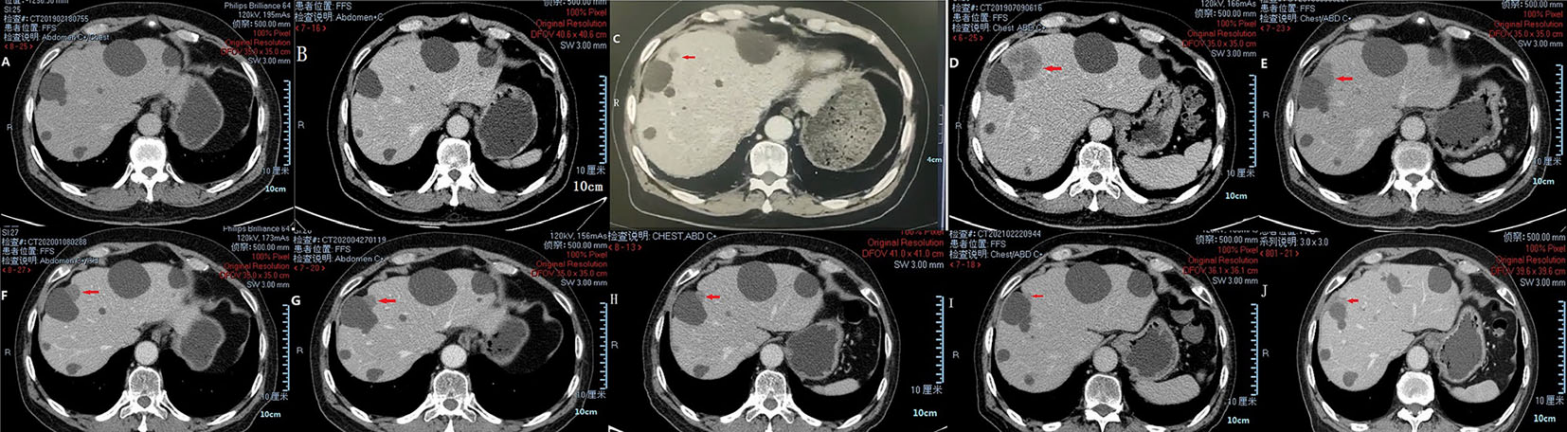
CT images of liver metastases during treatment of pembrolizumab and toripalimab combined with anlotinib. Multiple liver cysts (A, B). Multiple new lesions in the liver (C). Enlarged new liver lesions (D). Liver lesions controlled *via* treatment (E–J).
